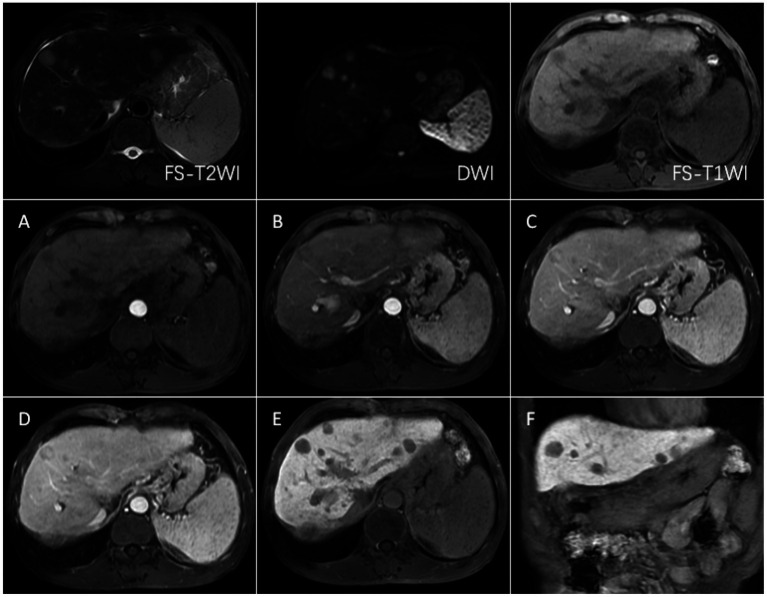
FS-T2WI and DWI: several round-like T2WI and DWI high-signal nodules were seen in the left and right lobes of the liver, with the largest being about 19.6 mm in diameter; FS-T1WI: several round-like low-signal nodules were seen in the left and right lobes of the liver. (A–F) show the early arterial, late arterial, portal venous, delayed, hepatobiliary axial and coronal positions. MRI dynamic scan: the lesion did not have definite enhancement in the early arterial stage, and it showed inhomogeneous and mild enhancement in the late arterial stage, with low-signal edges in the portal vein stage and the delayed stage, and high-signal in the central area; Hepatobiliary phase—axial and coronal: the lesion showed low signal, no contrast uptake was seen, suggesting that the multiple intrahepatic nodules were multiple HCC.
